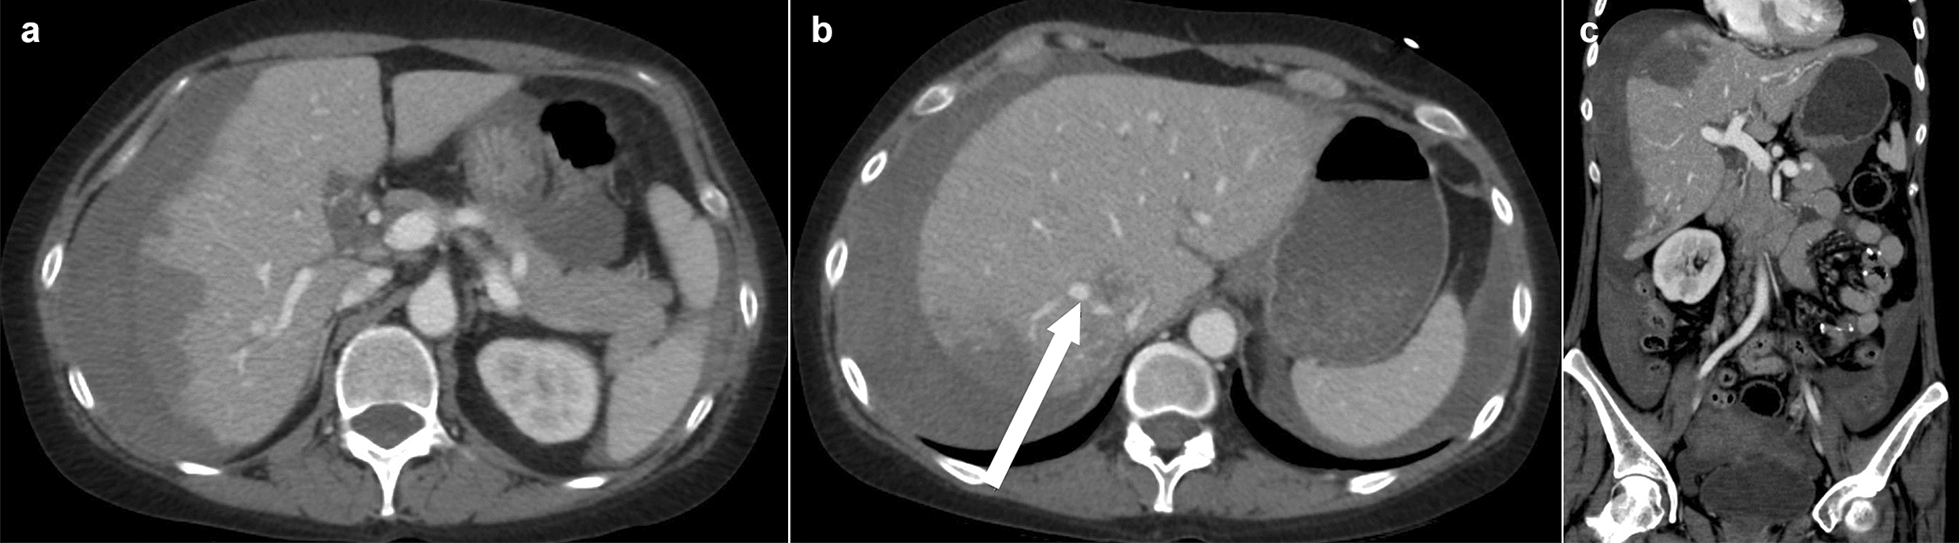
Abdomen-pelvis computed tomography with contrast. a Coronal view, demonstrating hepatic subcapsular hematoma in right lobe. b Coronal view. Arrow indicates suspicious aneurysmal change. c Sagittal reconstructed view, revealing massive amount of hemoperitoneum caused by suspicious aneurysmal rupture
